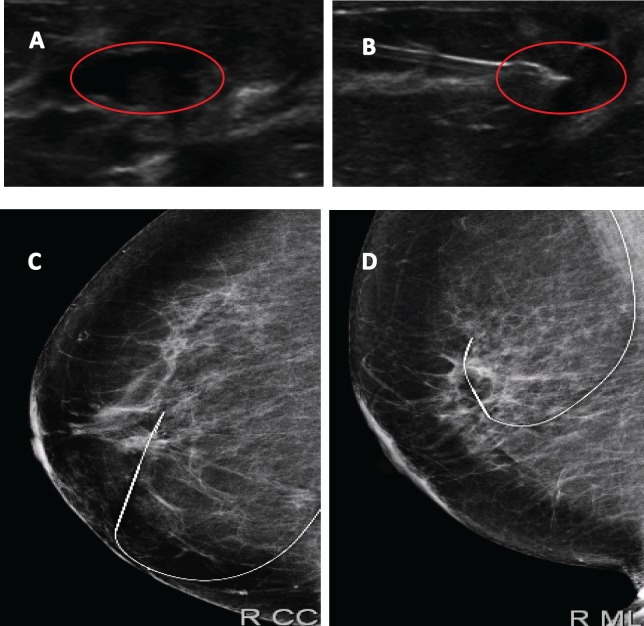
Image of papillary lesion and preoperative marking. A. Dilated milk duct with hypoechoic content. B. Marking on ultrasound lesion to be dried up. C and D Metal guide in mammographic projections LCC and LML.
